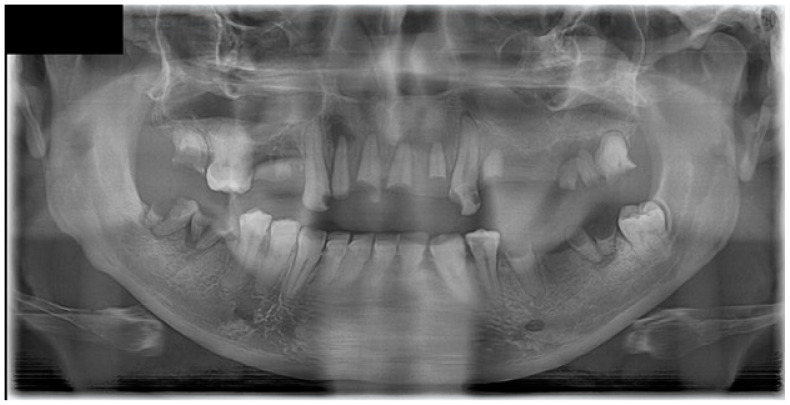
Panoramic radiograph (dental orthopantomogram, DOP) demonstrating extensive dental pathology and maxillary involvement. The image shows multiple residual roots, advanced carious destruction, generalized periodontal bone loss, and irregular osteolytic changes in the left maxillary alveolar process, corresponding to the clinically affected region. These findings initially supported an odontogenic and inflammatory etiology but did not fully explain the progressive, destructive soft tissue lesion.
